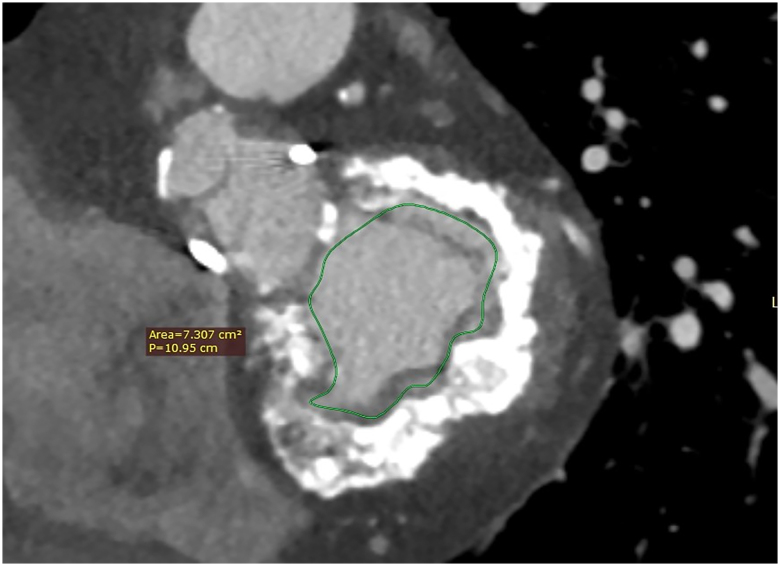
Initial cardiac computed tomography—mitral annular calcification. Cardiac computed tomography demonstrating the almost circumferential mitral annular calcification, as well as the mitral valve annular area. 100 × 138 mm.
